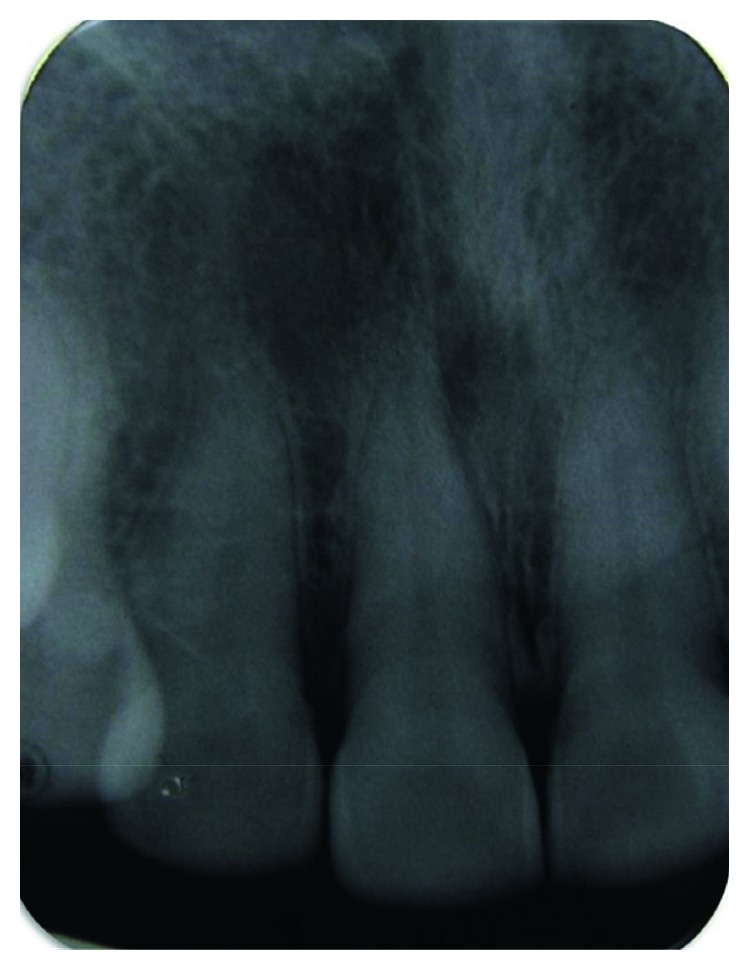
Intraoral periapical radiograph showing mild alteration in the trabecular pattern in relation to 11 and 12.
